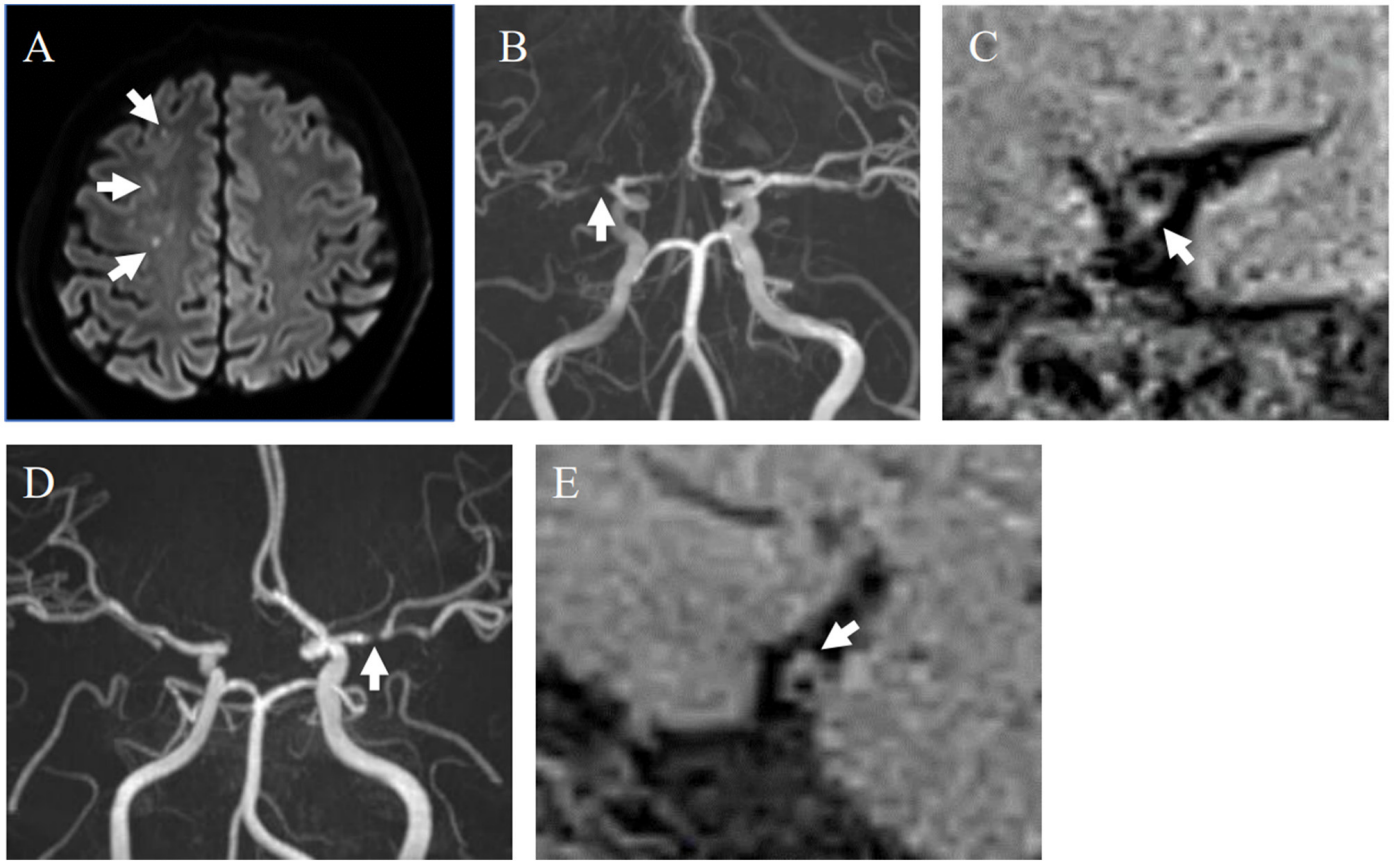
Representative cases with and without HIPs. (A–C) A 40-year-old male with symptomatic stenosis in the right MCA. (A) Multiple cerebral infarcts were detected in the right cerebral white matter on DWI (white arrows). (B) MRA showed severe stenosis of the M1 segment of the right MCA (white arrow). (C) An HIP was detected on the sagittal section of the MCA on DANTE T1-SPACE (white arrow). SIplaque/SImuscle ratio was 1.61. This case was diagnosed as A-to-A embolism. (D, E) 59-year-old female with asymptomatic stenosis in the left MCA. (D) MRA showed severe stenosis of the M1 segment of the left MCA (white arrows). (E) The plaque without hyperintensity was detected on the sagittal section of the MCA on DANTE T1-SPACE. SIplaque/SImuscle ratio was 1.33.
